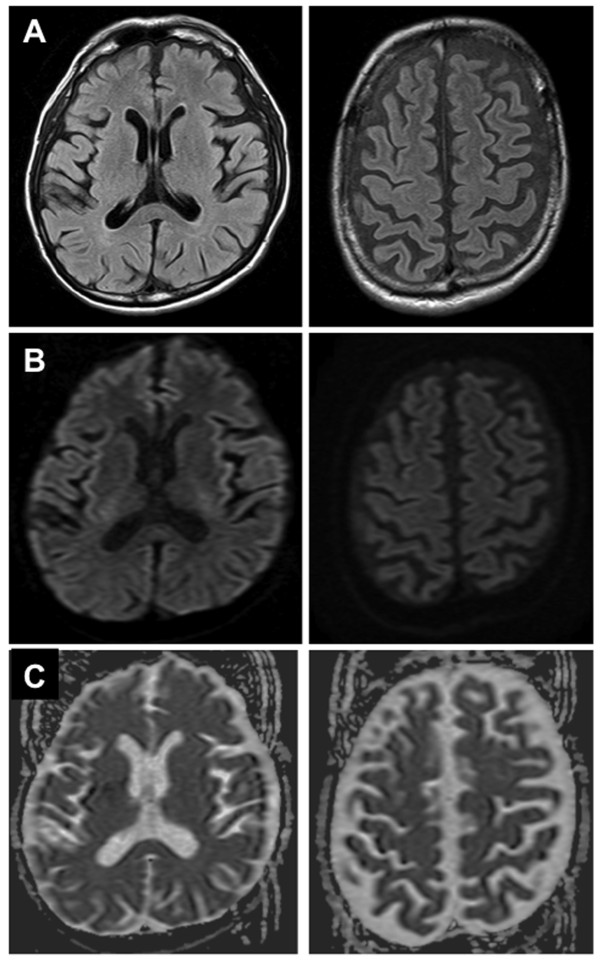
MRI after therapy. Representative FLAIR images (A), DWI (B), and ADC map (C) 17 days after admission, showing disappearance of the hyperintense lesions.
